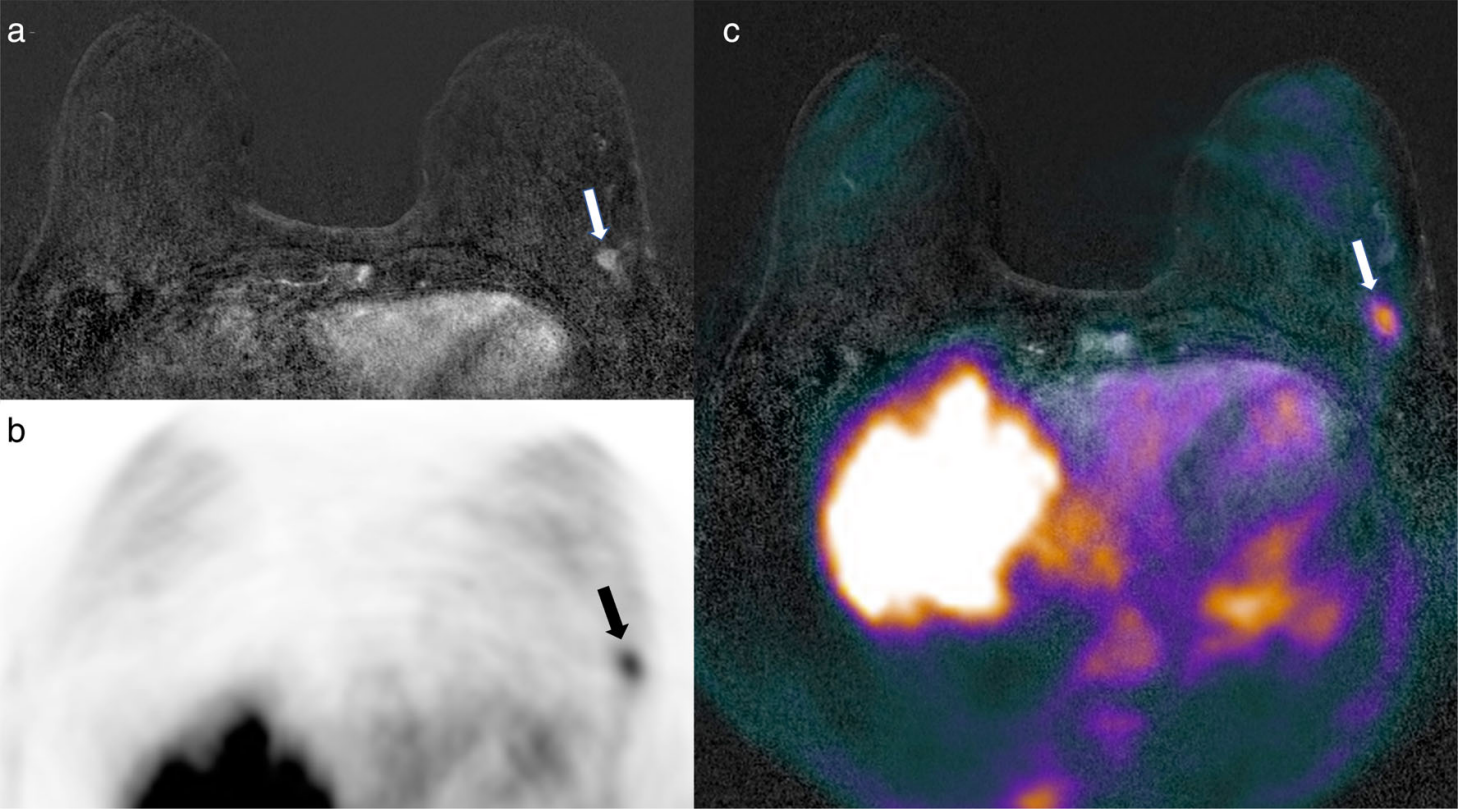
Axial (a) subtracted dynamic contrast‐enhanced (DCE)‐MRI, (b) PET, and (c) fused DCE‐MRI and PET imaging. A 66‐year‐old patient with invasive ductal breast cancer (9 mm, G3, ER/PgR−, HER2+) of the left breast (arrows in a–c).
